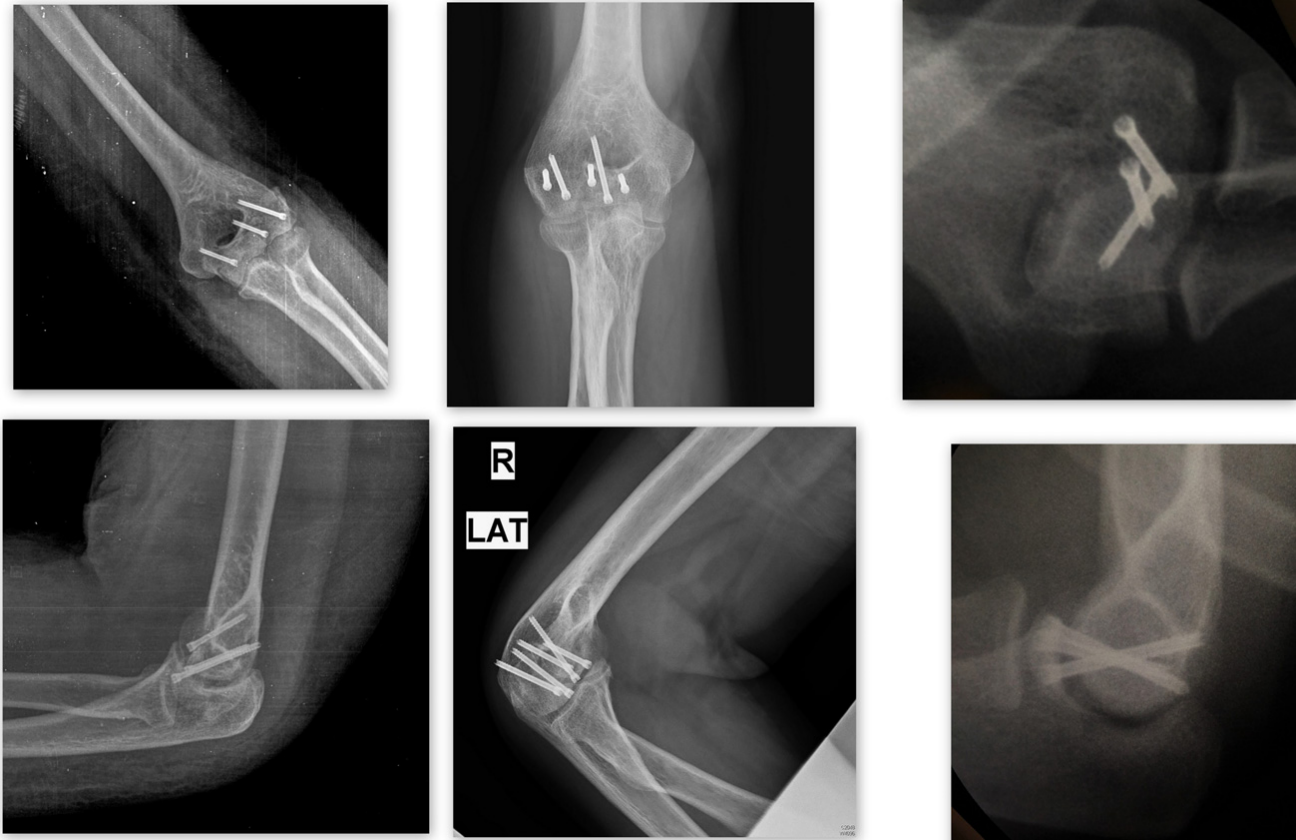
Post op X-rays showing union of fracture.
